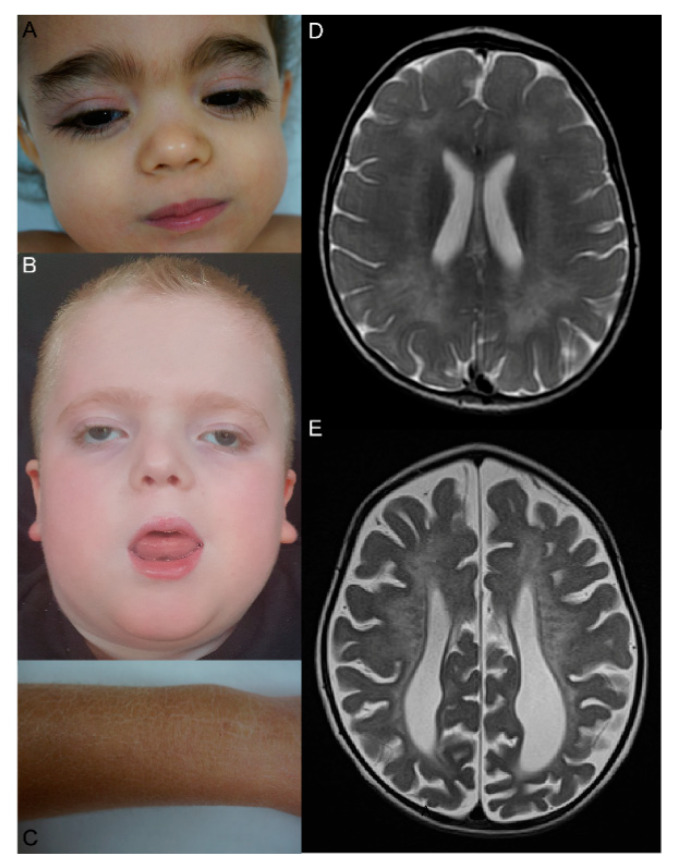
(A,B): Facial appearance of multiple sulfatase deficiency (MSD) patients. (C): Ichthyosis in a MSD patient. (D,E): T2-weighted cMRI pictures from two different MSD patients displaying typical signs of a leukodystorphy with radiating stripes (D) and additional progressive cerebral atrophy (E).
